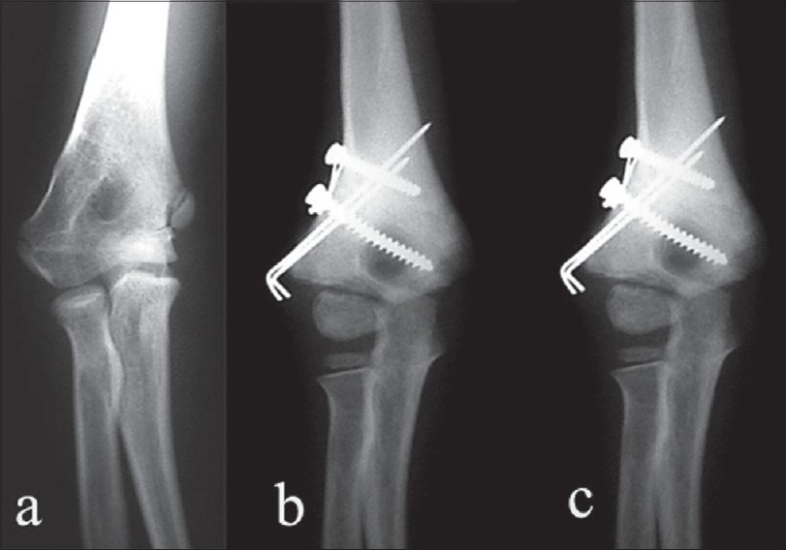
Radiograph (anteroposterior view) (a) Preoperative showing cubitus varus deformity. (b) Three months postoperative showing maintenance of correction and normal valgus achieved. (c) Eight months postoperative showing maintenance of correction.
